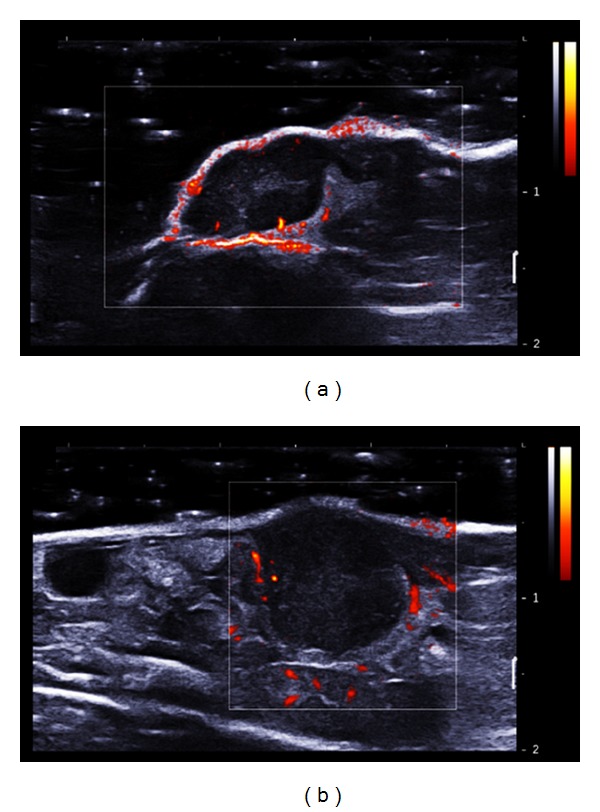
Visualization of tumour vascularization with Doppler mode (standard Doppler fixed between 0 and 2 m/s). (a) CT26 ectopic tumour and (b) orthotopic CT26 tumour 15 days after tumour implantation. The blood velocity was color coded in scales ranging from 0 to 2 m/s.
